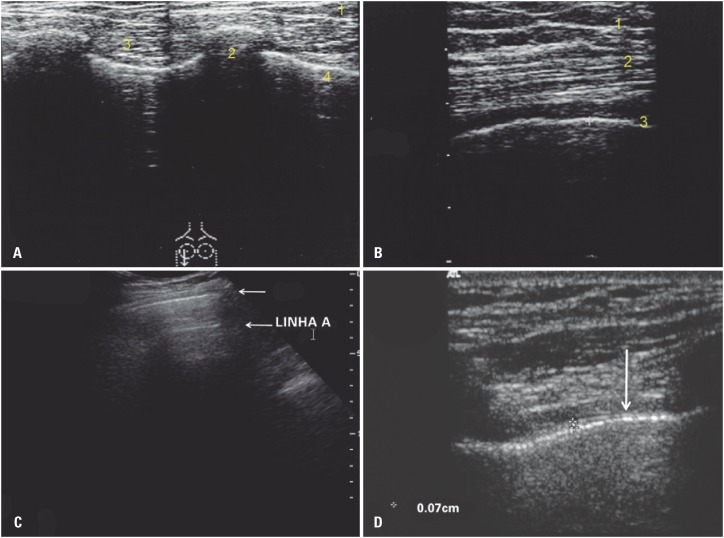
Sonographic anatomy. (A) Chest wall sonogram (7 MHz linear probe). (1) Observe the different tissue planes displayed: skin and subcutaneous tissue, (2) ribs (transverse plane), (3) intercostal muscles, (4) pleural line. (B) Sonographic anatomy of the chest wall (7 MHz linear probe). (1) Observe the different tissue planes displayed (from the surface down): skin and subcutaneous tissue, (2) pectoral muscles note the typical fibrillar appearance and the, (3) pleural line. (C) Echogram pattern of the normal pleura (3.5 MHz convex probe). Only the pleural line can be identified at this frequency; the parallel subpleural A line is enhanced (reverberation artifact). Low image resolution interferes with recognition of pleural line layers. (D). Pleural line. Both portions of the pleura (echoic lines indicated by measuring devices) and the anechoic space between them are displayed. The visceral and parietal pleuras correspond to the inner and outer echoic lines, respectively; the extrapleural hypoechoic line (arrow) corresponds to extrapleural fat. Note big image resolution differences; higher image resolution achieved with higher frequency probes enables detailed visualization of pleural line components
